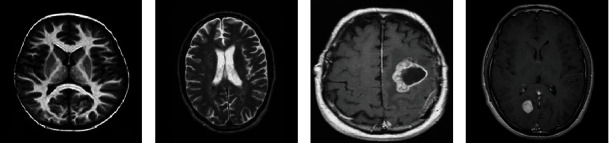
BR253 MRI image: (a, b) nontumorous brain and (c, d) tumorous brain.
